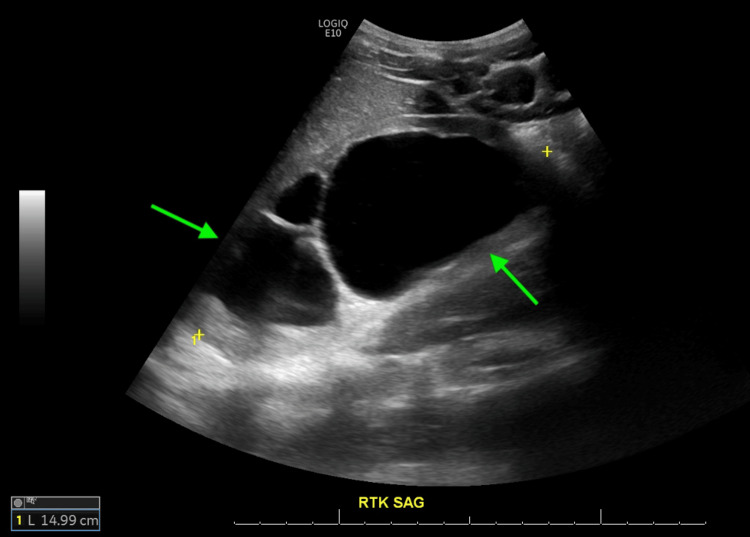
Left-sided renal ultrasound shows severe hydronephrosis with cortical thinning and the presence of echogenic material in renal pelvises, along with proximal ureters.
